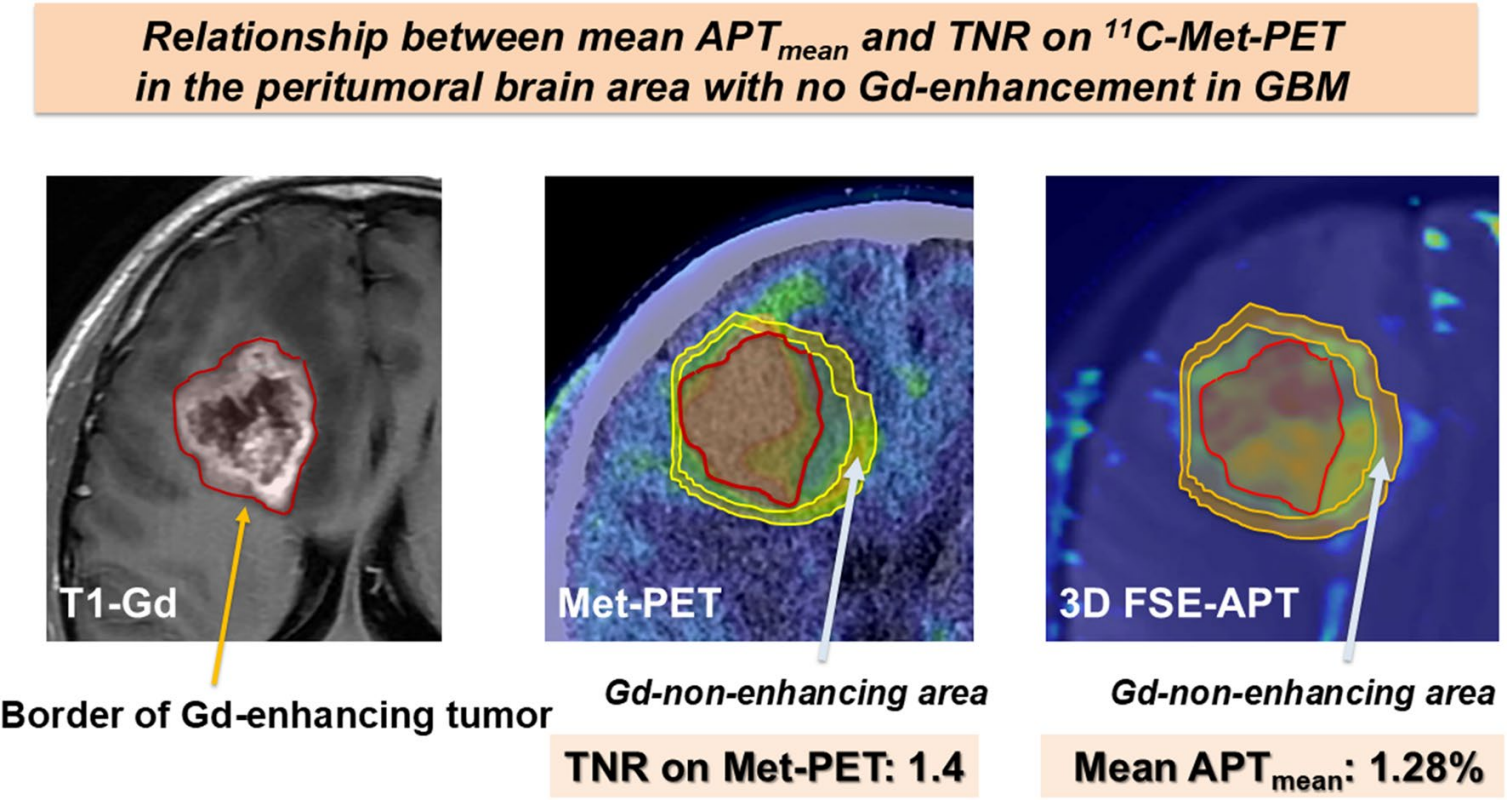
Relationship between MRI (T1-gadolinium (Gd)), ^11^C-methionine (Met)-positron emission tomography (PET) and mean APT_mean_ for GBM, IDH wild type. Mean APT_mean_ for APT imaging by 2D SSFSE acquisition with CEST at the site of tumor-to-contralateral normal brain tissue ratio (TNR) 1.4 for Met accumulation rate in ^11^C-Met-PET is 1.52 ± 0.16%. The cut-off for mean APT_mean_ to optimally distinguish TNR < 1.4 for ^11^C-Met-PET is 1.28%, offering 100% sensitivity and 100% specificity according to receiver operating characteristic (ROC) analysis. Red area: border of gadolinium (Gd)-enhancing tumor. Yellow area: TNR of 1.4 on ^11^C-Met-PET. Orange area: mean APT_mean_: 1.28%
